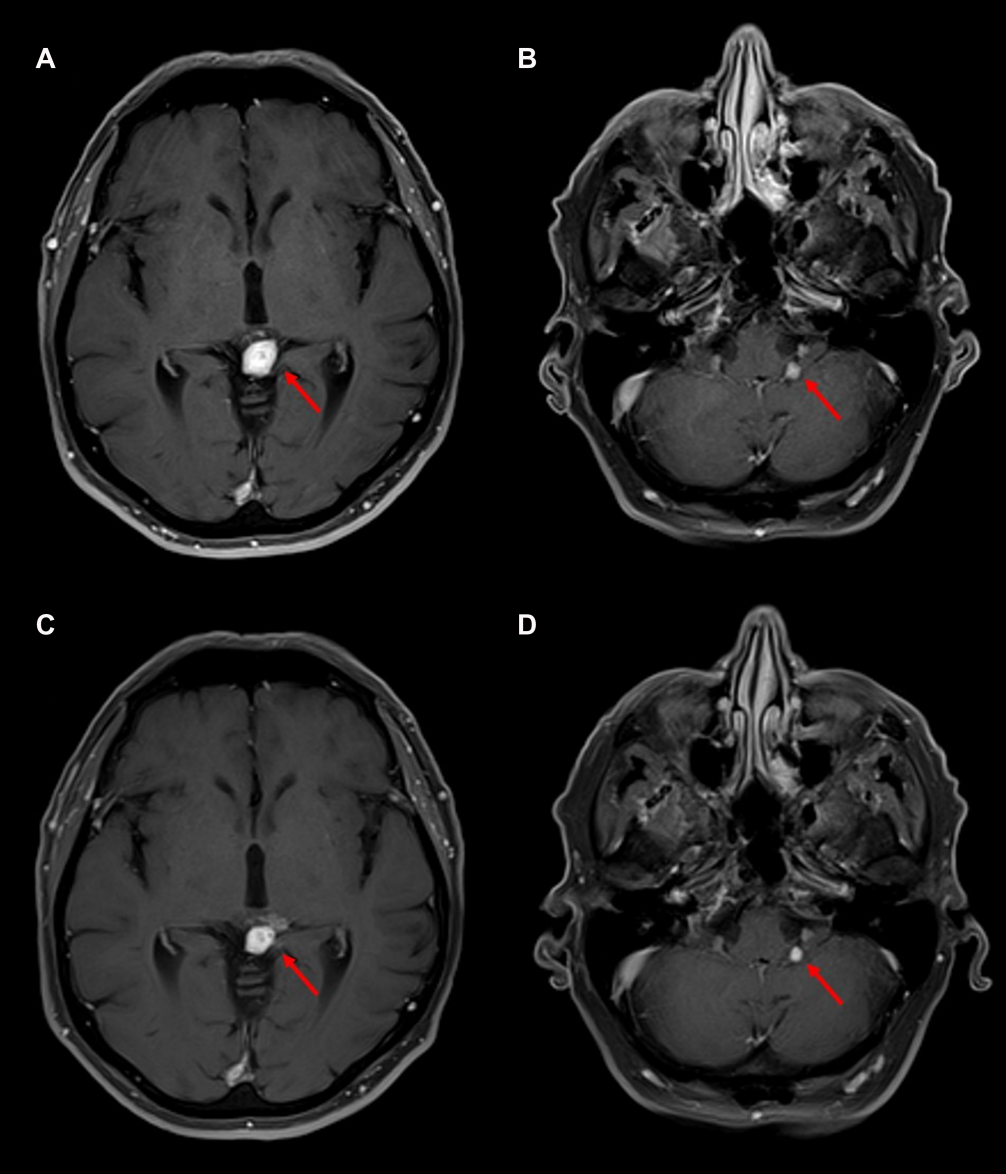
Follow-up MRI brain scans after four cycles of ^177^Lu-dotatate. T1-post-gadolinium sequences are shown. (A) MRI at 47 months after diagnosis, four months after completing ^177^Lu-dotatate, showing a 1.4 cm × 1.0 cm enhancing pineal mass, slightly decreased in size from diagnosis. (B) MRI 4 months after ^177^Lu-dotatate showing a 0.4 cm × 0.4 cm unchanged left cerebellopontine angle lesion. (C) MRI at 55 months after diagnosis, 12 months after completing ^177^Lu-dotatate, showing a 1.0 cm × 0.9 cm enhancing pineal mass, decreased in size but with some increased minimally enhancing cystic components adjacent but not extending from the pineal mass. (D) MRI 12 months after completing ^177^Lu-dotatate showing a 0.4 cm × 0.4 cm left cerebellopontine angle lesion, largely unchanged in size.
